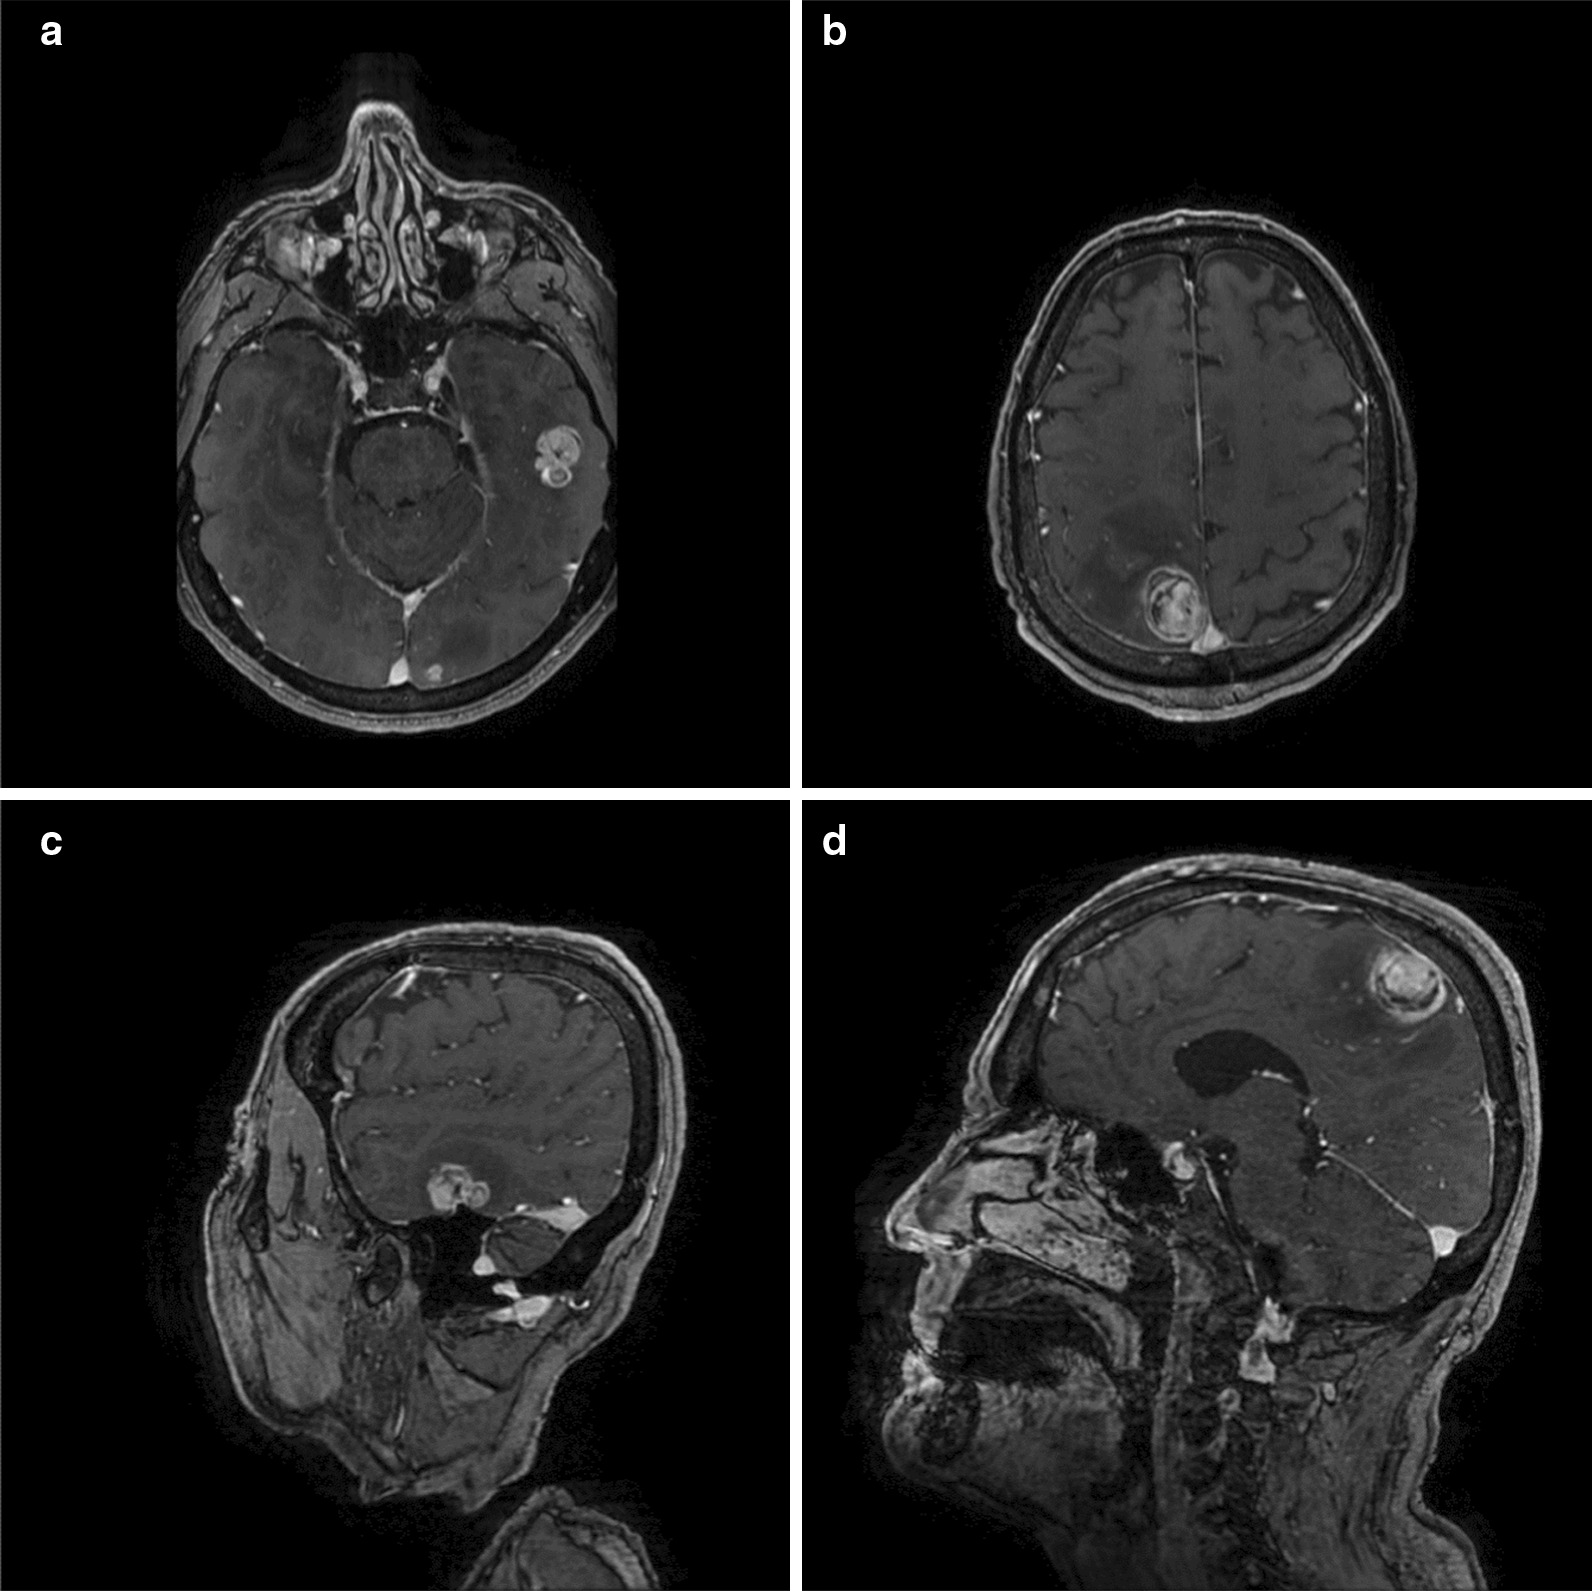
Contrast-enhanced T1-weighted magnetic resonance imaging scans demonstrating left temporal (a, c) and right parietal (b, d) metastases of leiomyosarcoma (a+b axial; c+d: sagittal)
